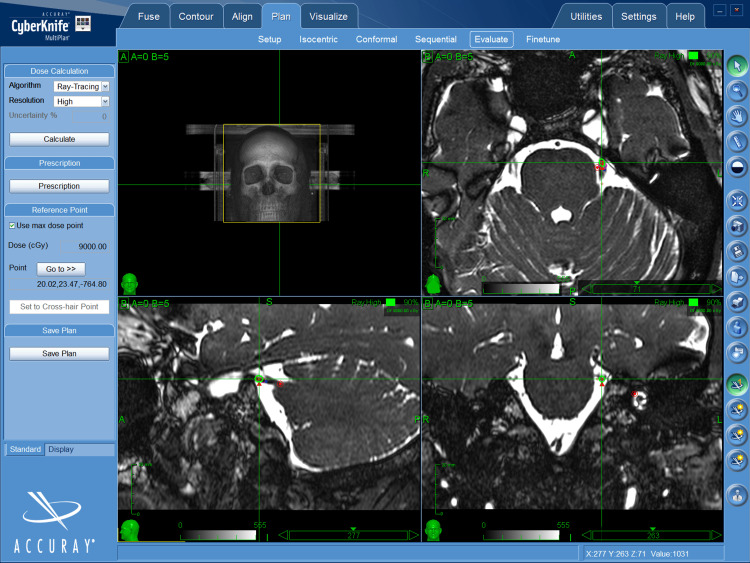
MRI fast imaging employing steady-state acquisition (FIESTA) sequence showing the trigeminal nerve at the level of the cisternal segment of the posterior fossa. The dose and treatment point was located 4-5 mm from the apparent origin of the trigeminal nerve or dorsal root entrance zone (DREZ).
